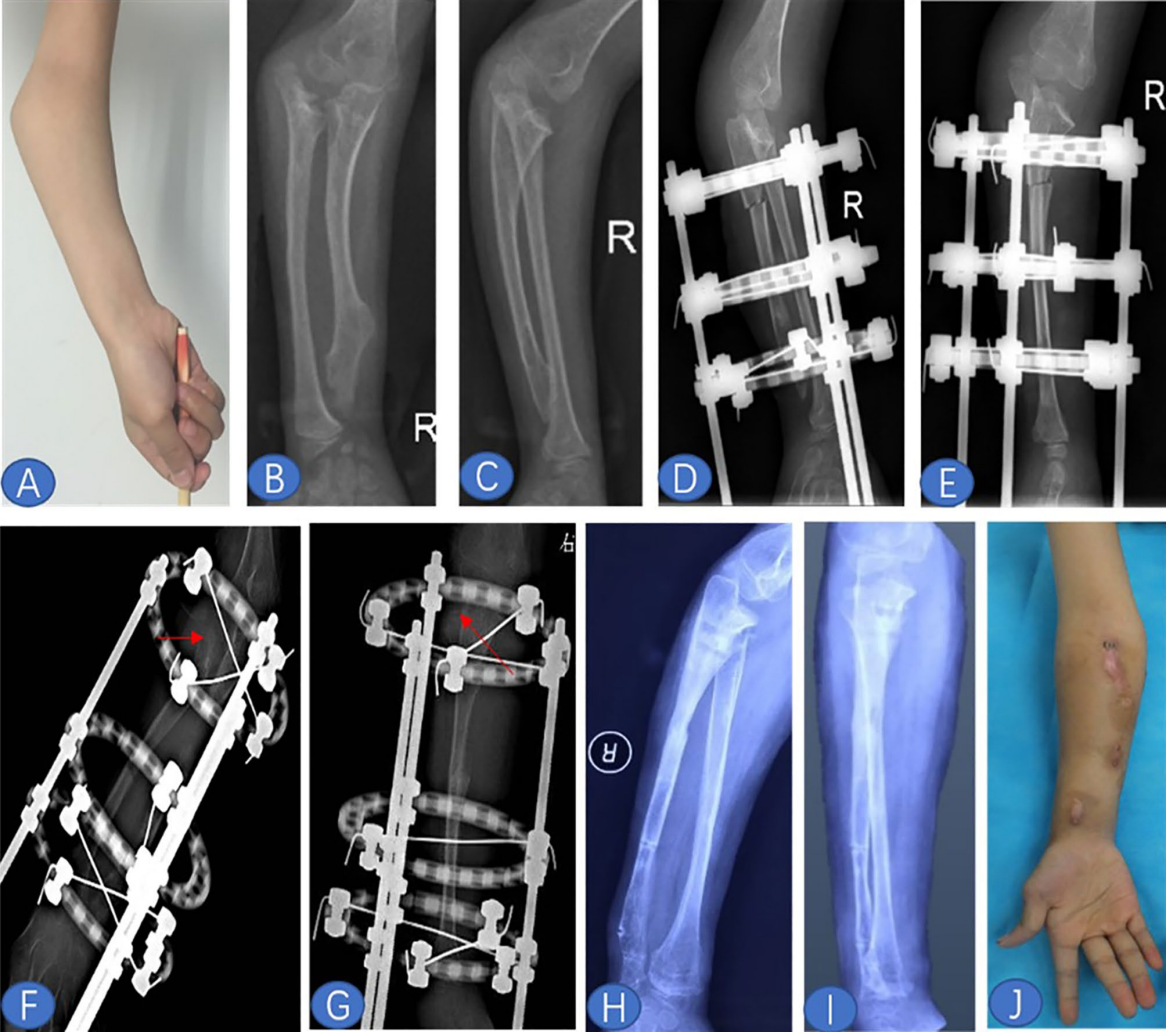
Functional and cosmetic improvement were achieved in all cases. Preoperative cosmetic (A) and radiological (B, C), the anteroposterior (D) and lateral (E) view X-ray of the forearm on the first day after operation, the radial head distracted at the same level with the coronoid process (red arrow in F, G) adjust the K-wire (remove the distal radius fixation and fix the proximal radioulnar joint), and postoperative radiological (H, J) and cosmetic (J) views of case 5.
